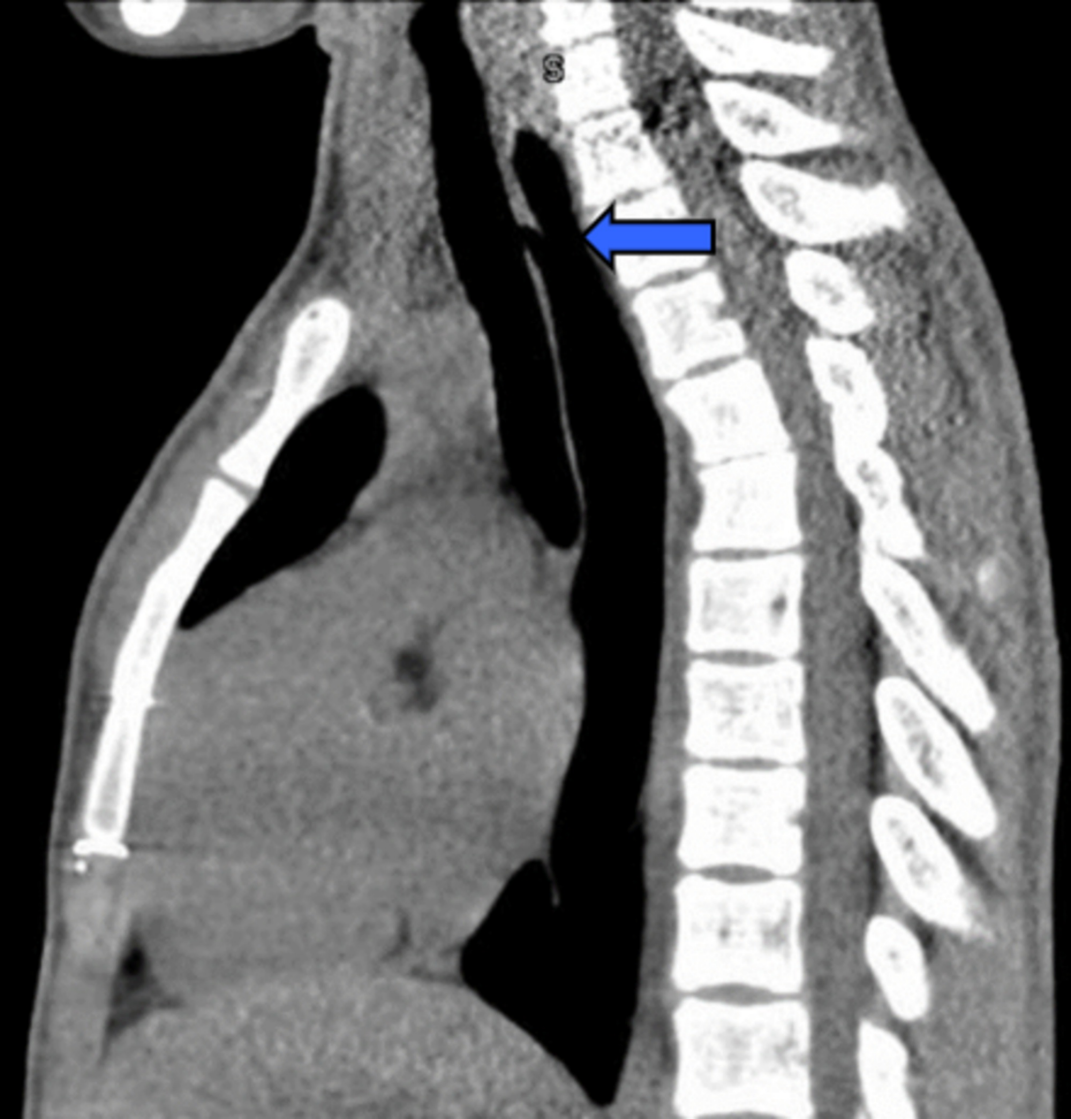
CT scan of chest without contrast, sagittal view, demonstrating TEF (blue arrow). TEF: tracheoesophageal fistula.
